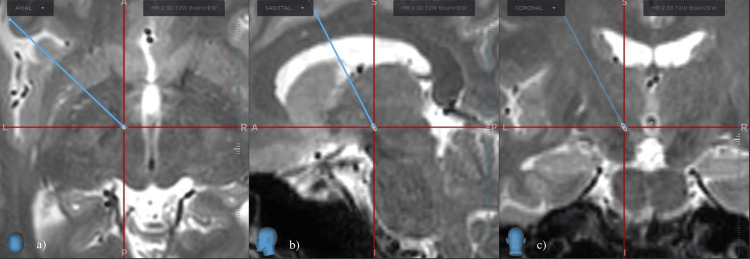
Preoperative MRI Preoperative MRI T2-weighted imaging with (a) axial, (b) sagittal, and (c) coronal views. The blue line denotes the planned trajectory, while the intersection of the red lines represents the planned target.
